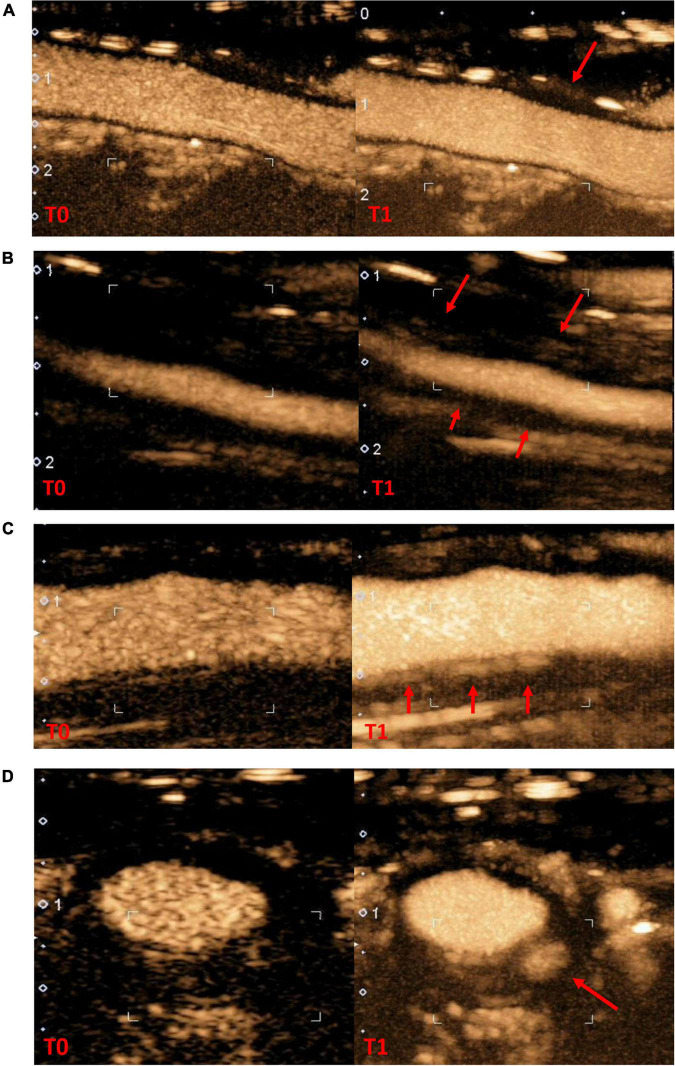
Contrast-enhanced ultrasonography in arterial wall in giant cells arteritis patients (A), no contrast-enhanced (B), grade 1 moderate contrast-enhanced (C), grade 2 high contrast-enhanced in longitudinal section (D), grade 2 contrast-enhanced in cross section (T0: wall reference at the start of contrast infusion; T1: wall contrast at the end of the recording, red arrow specific arterial wall thickening area where contrast-enhanced was evaluated).
